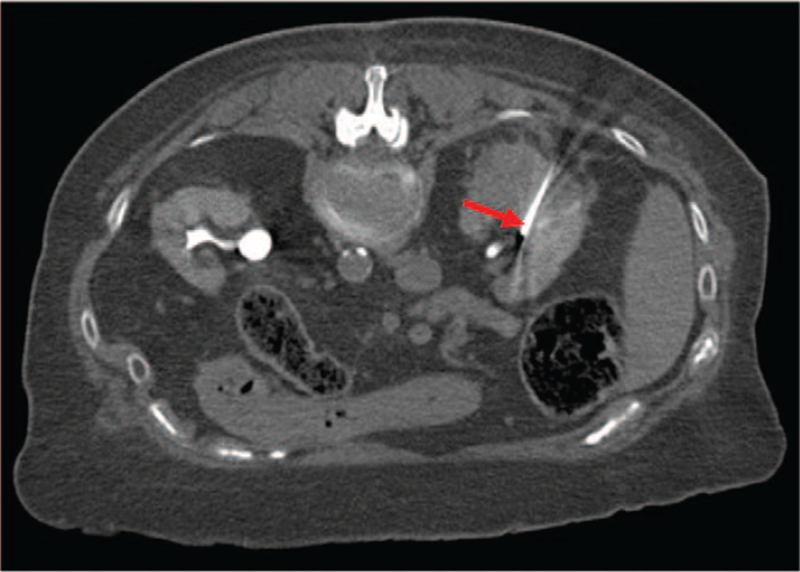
Abdomen CT scan during ECT treatment: the red arrow indicates one of the needle electrodes inserted into the residual lesion.
